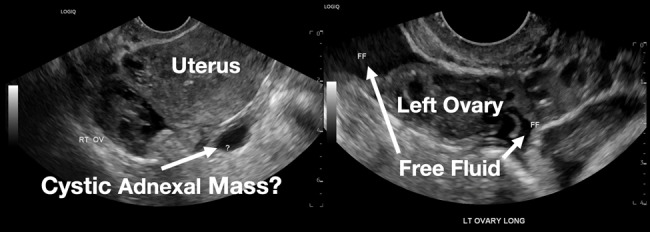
This image shows the patient’s ultrasound findings 11 days after her initial diagnosis of a left tubal pregnancy. The image on the left shows the right adnexa with an arrow pointing to a rounded cystic structure with a peripheral soft tissue component, blood flow, and free fluid. The image on the right shows the left ovary with two arrows pointing to a moderate amount of free fluid in the left adnexa.
